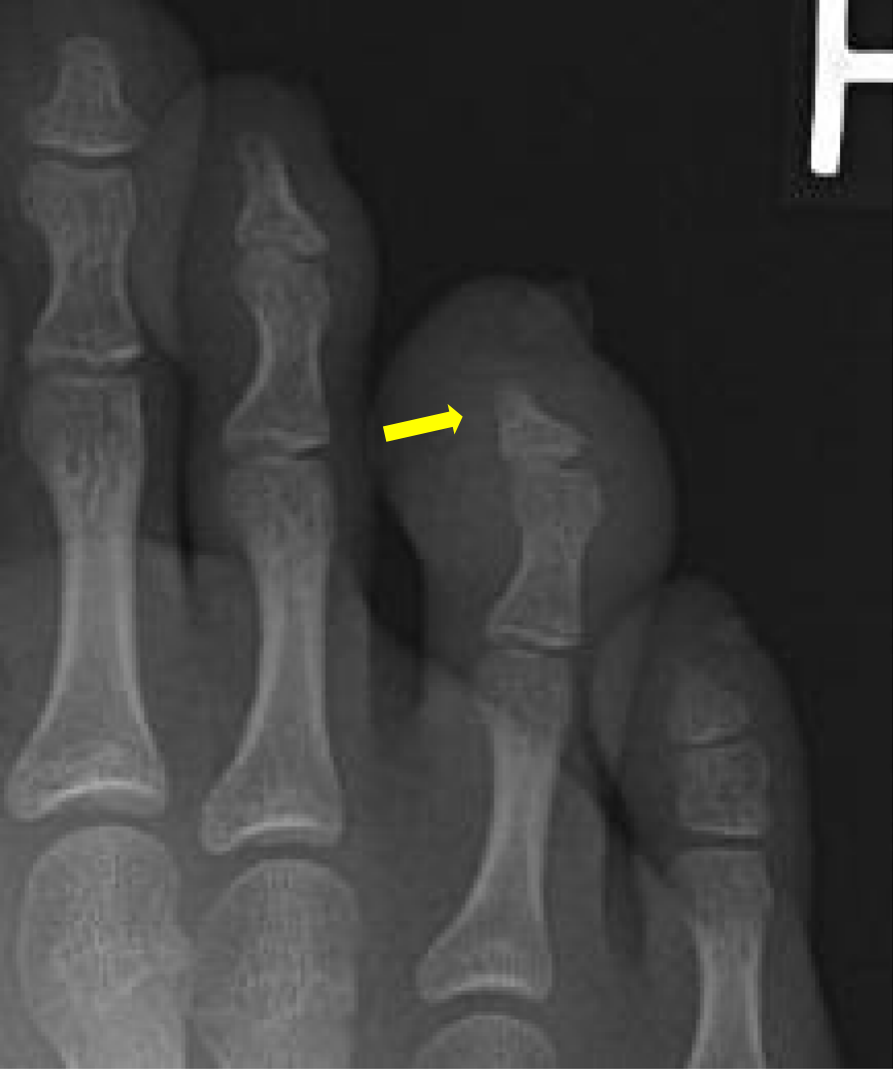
Frontal radiograph of the toes in a 14 year old female patient with juvenile psoriatic arthritis demonstrates focal soft tissue swelling of the fourth digit, with tapering of the distal phalanx and resorption of the distal tuft cortex
